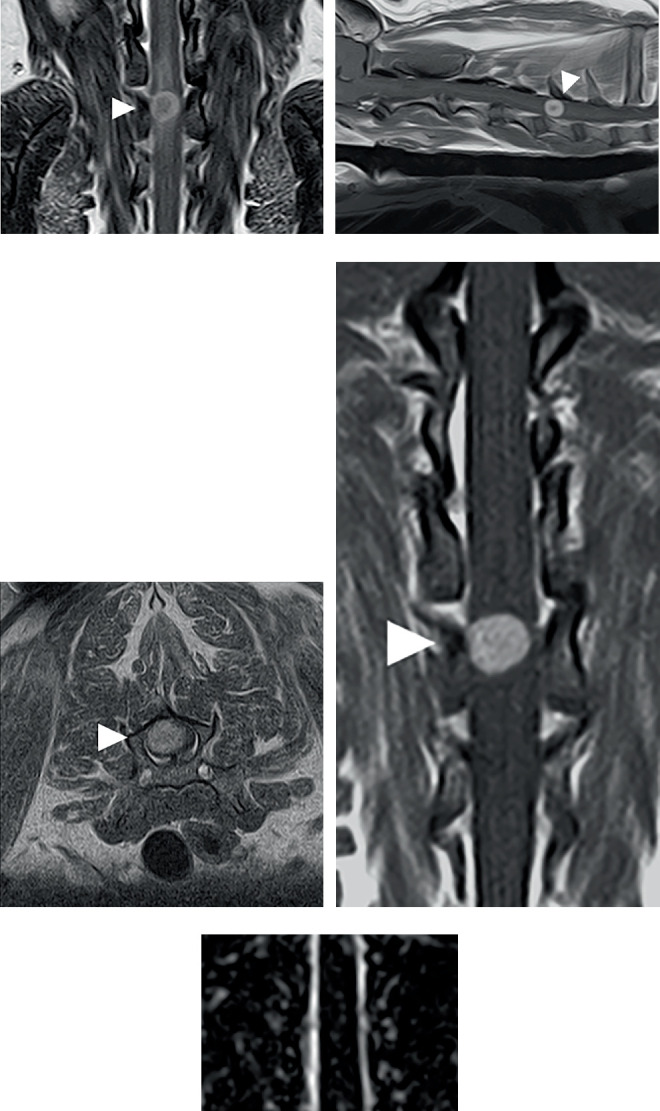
Magnetic resonance imaging (MRI) images of the hemangioblastoma. T2-weighted MRI images in the (a) sagittal, (b) transverse, and (c) dorsal plane. Contrast-enhanced T1-weighted MRI images in the (d) sagittal, (e) transverse, and (f) dorsal plane. (g) MR myelography image in the dorsal plane. White arrowheads refer to the mass.
